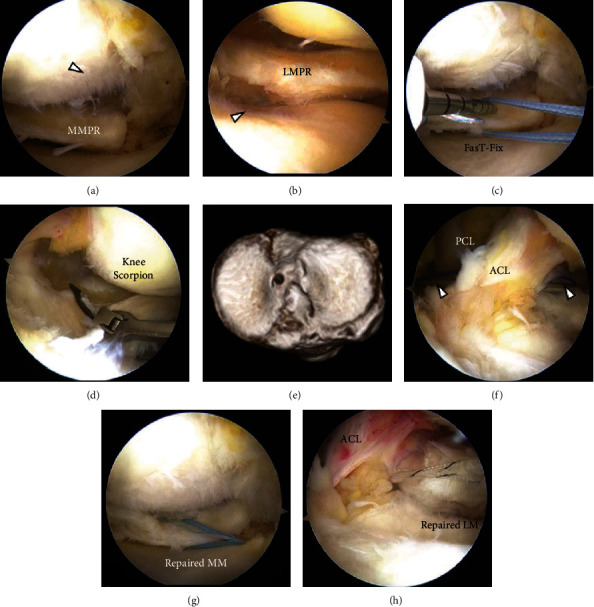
Arthroscopic findings and three-dimensional computed tomography. (a) Medial meniscus posterior root tear and highly damaged articular surface on the medial femoral condyle (arrowhead). (b) Lateral meniscus posterior root tear and highly damaged articular surface on the lateral tibial plateau (arrowhead). (c) FasT-Fix (Smith & Nephew, Andover, MA, USA) dependent modified Mason-Allen suture technique. (d) A simple stitch suture using the Knee Scorpion suture passer (Arthrex, Naples, FL, USA). (e) Position of the tibial tunnel. (f) Suture is passed between the ACL and the PCL using a suture retriever (Smith & Nephew) (arrowhead). (g) Repaired MM. (h) Repaired LM. MM: medial meniscus. LM: lateral meniscus. ACL: anterior cruciate ligament. PCL: posterior cruciate ligament. MMPR: medial meniscus posterior root. LMPR: lateral meniscus posterior root.
